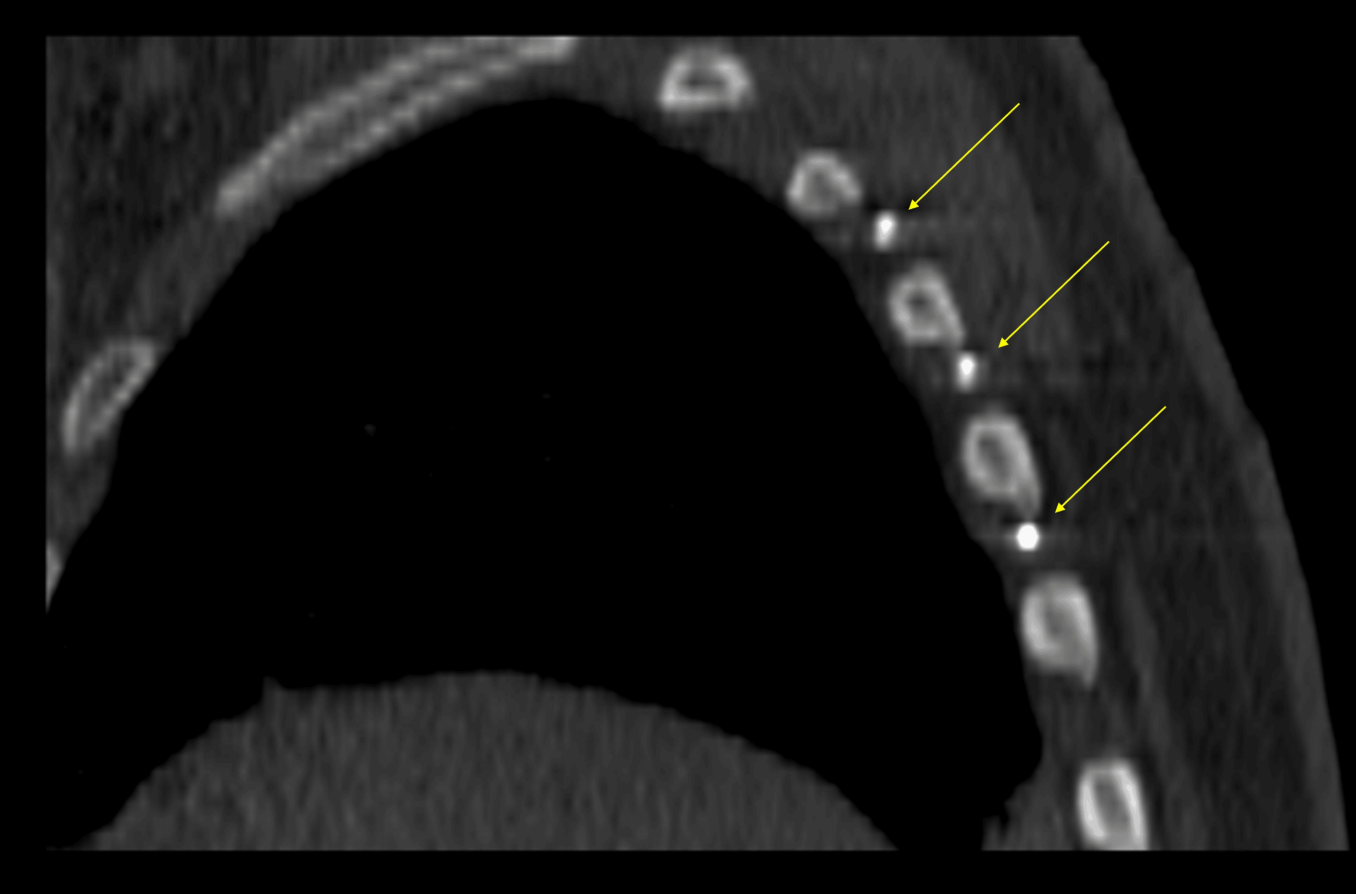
Intraprocedural sagittal computed tomographic image showing three cryoablation probes (arrows) within T4-T7 intercostal spaces
